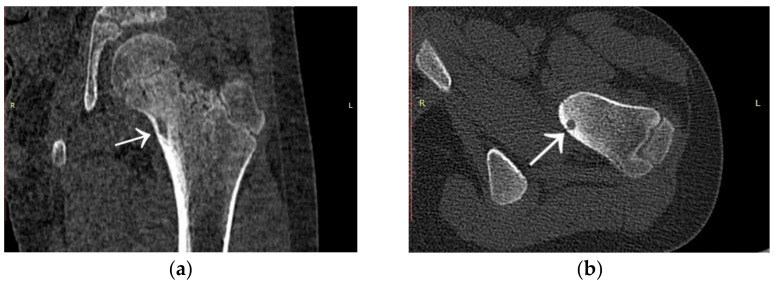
CT scans showing a focus of OO (arrows) in the left femoral neck. (a) Frontal CT scan; (b) Transverse CT scan.
